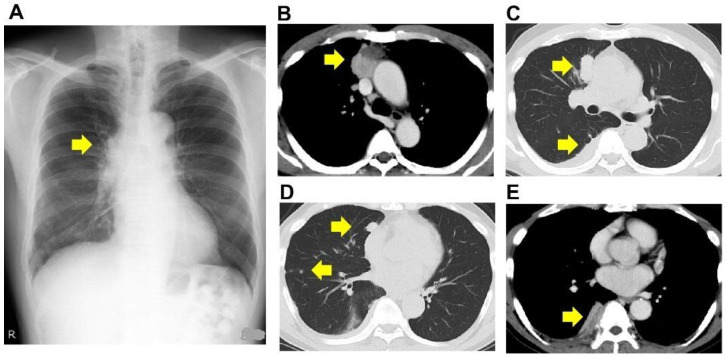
Radiological findings. Plain radiograph (A) and computed tomography (B–E). The computed tomography revealed a tumor between the anterior mediastinum and the right lung upper lobe (B,C), multiple nodular shadows along the right pleura (D), and pleural effusion (E). Arrows indicate the lesion.
